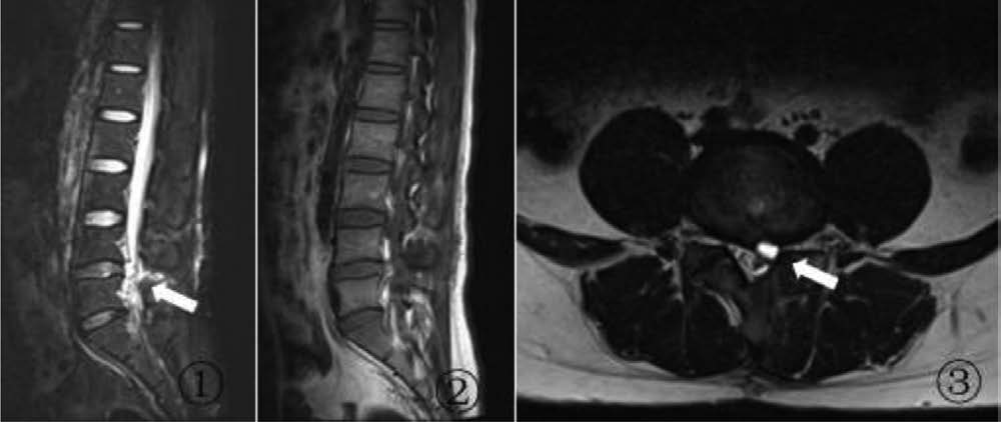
MRI of the lumbar spine after lumbar discectomy. A compressive cystic lesion with (A) high signal intensity on a sagittal T2-weighted image, (B) low signal intensity on a sagittal T1-weighted image, and (C) high signal intensity on a transverse T2-weighted image is seen at the site of the previous lumbar discectomy surgery. MRI = magnetic resonance imaging.
